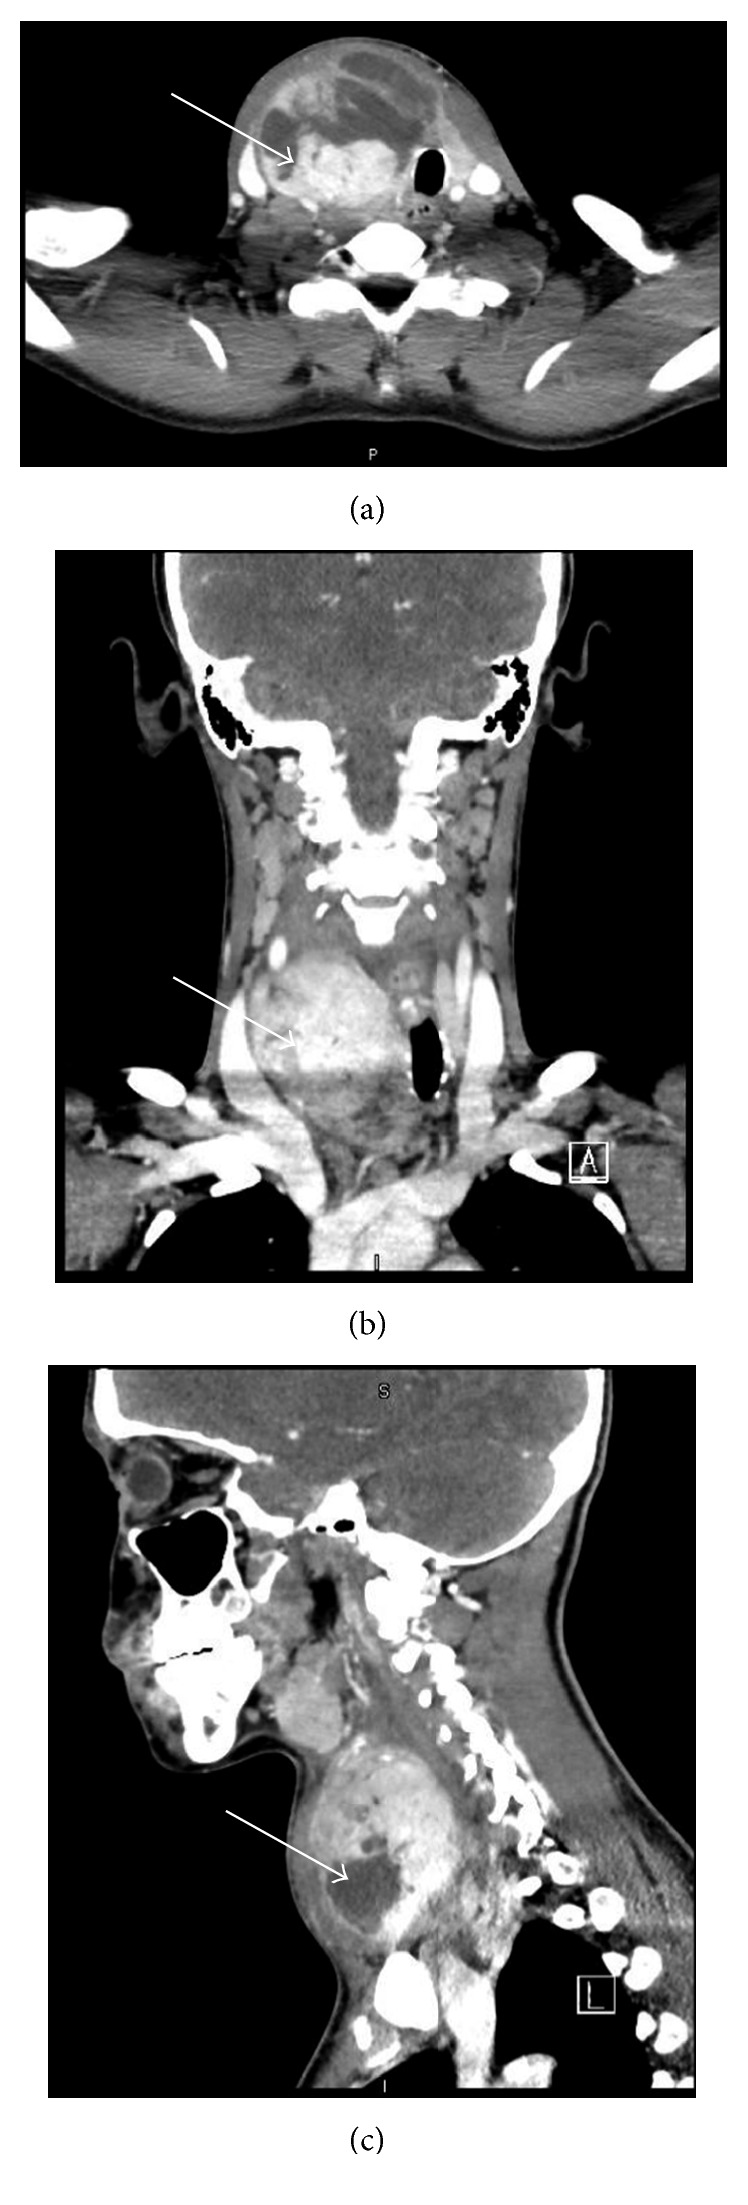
Computed tomography with contrast on admission showed a 6.4 × 6.1 × 7.1 cm heterogeneous mass with mild-to-moderate edema/inflammatory changes and mass effect on the trachea, right internal jugular vein, and right common carotid artery. (a) Cross-sectional, (b) coronal, and (c) sagittal sections.
